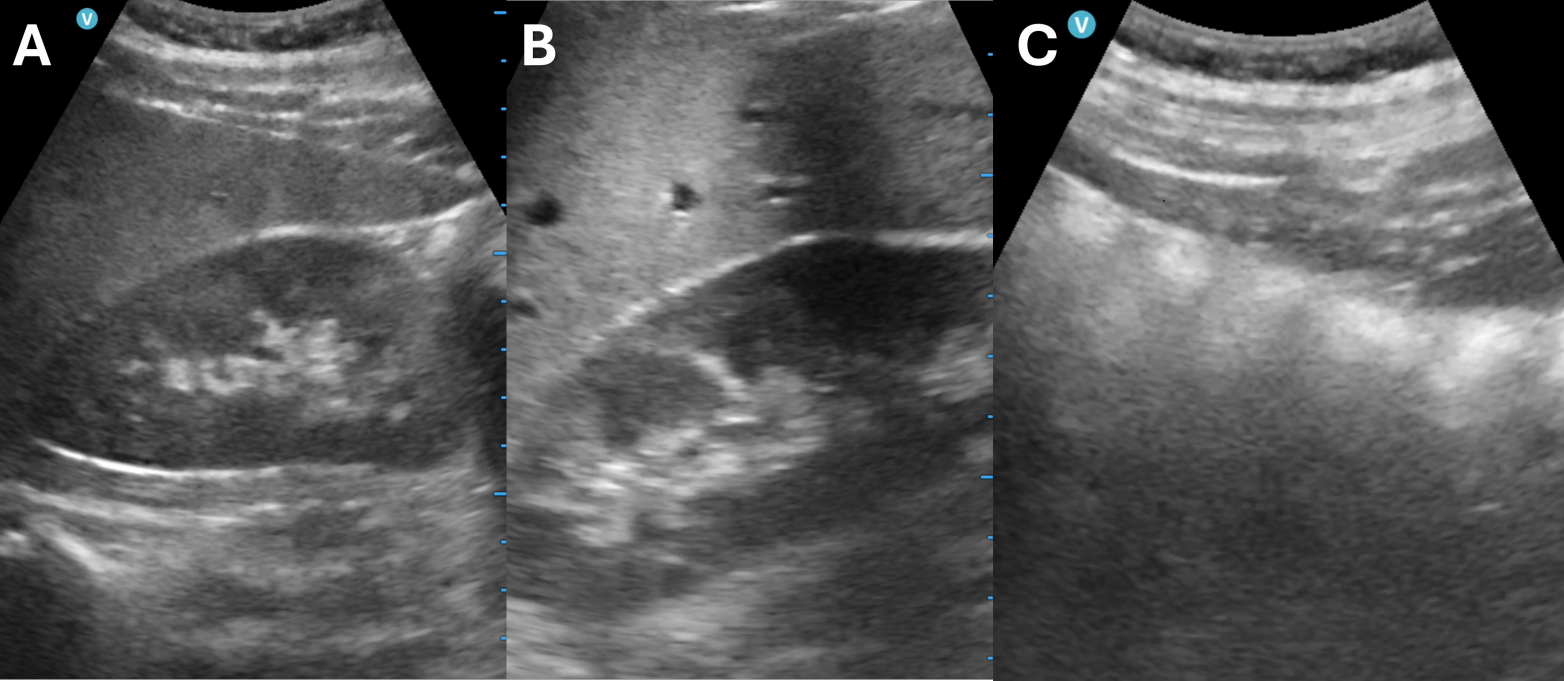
Examples of renal ultrasound images for model training: (A) optimal, (B) suboptimal (artifact and incorrect positioning), and (C) incorrect.
